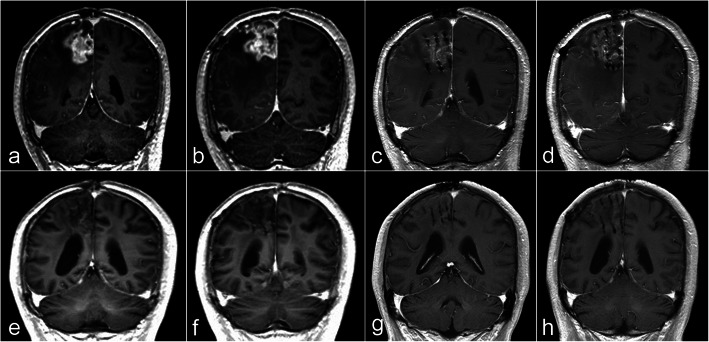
Postoperative follow-up of the case shown in Fig. 2 by MRI. a, b MR images before ^125^I brachytherapy treatment. c, d Enhanced T1W images 2 months after ^125^I brachytherapy shows that the enhancement of the tumor decreased significantly. e, f T1W images 6 months after brachytherapy shows that the enhancement of the tumor further decreased. The brain edema around the tumor was significantly decreased. g, h T1W images ten months after brachytherapy shows that there is no obvious enhancement of the tumor, which indicates that the tumor is well-controlled
